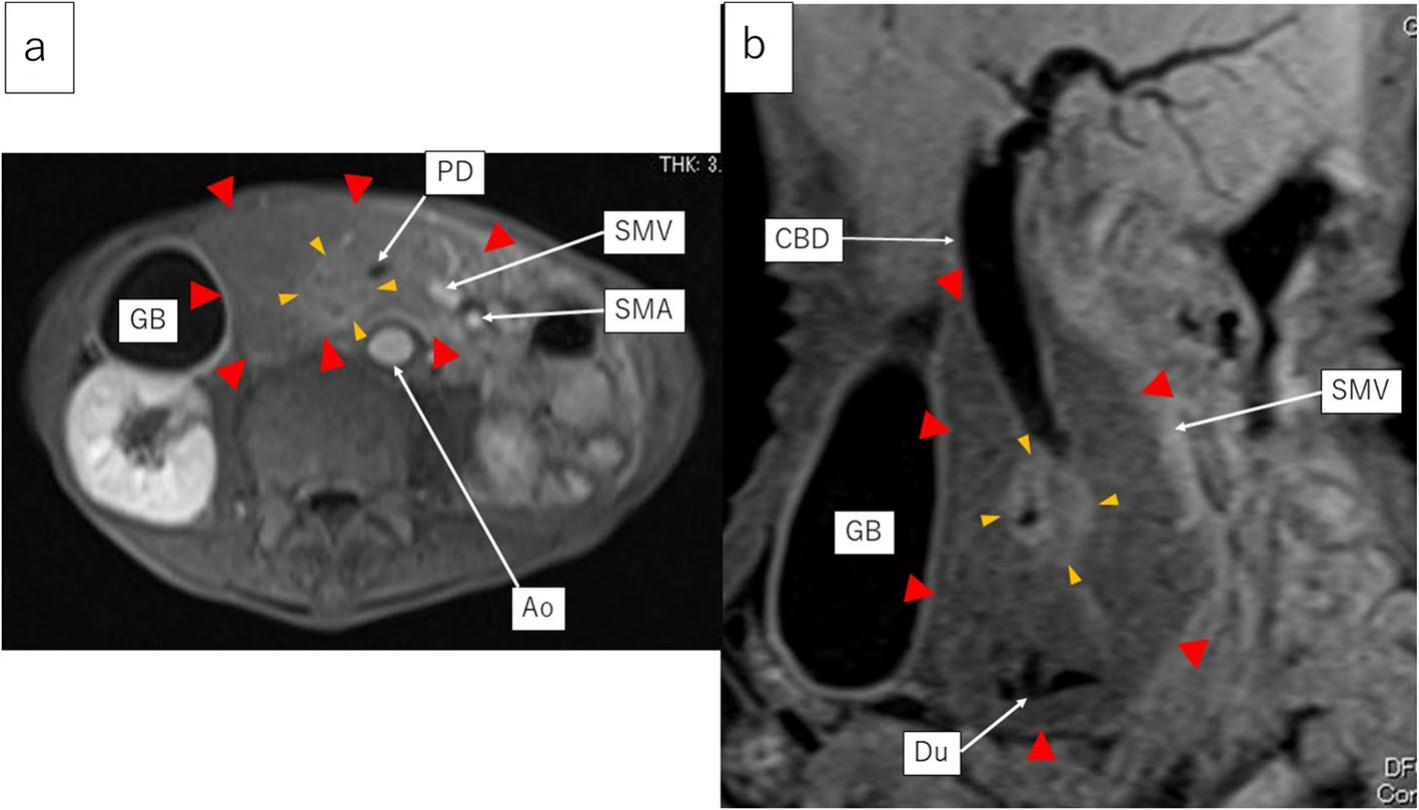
The late phase of contrast-enhanced MRI; a Axial view. b Coronal view. The peripheral part of the lesion had a low signal, while its central part had a high signal. The lower bile duct and the main pancreatic duct were involved and obstructed by the central part of the lesion. However, the upper and middle bile duct, superior mesenteric vein, duodenum, and gall bladder were not involved by the peripheral part of the lesion. Ao aorta, CBD common bile duct, Du duodenum, GB gall bladder, SMA superior mesenteric artery, SMV superior mesenteric vein, PD pancreatic duct
